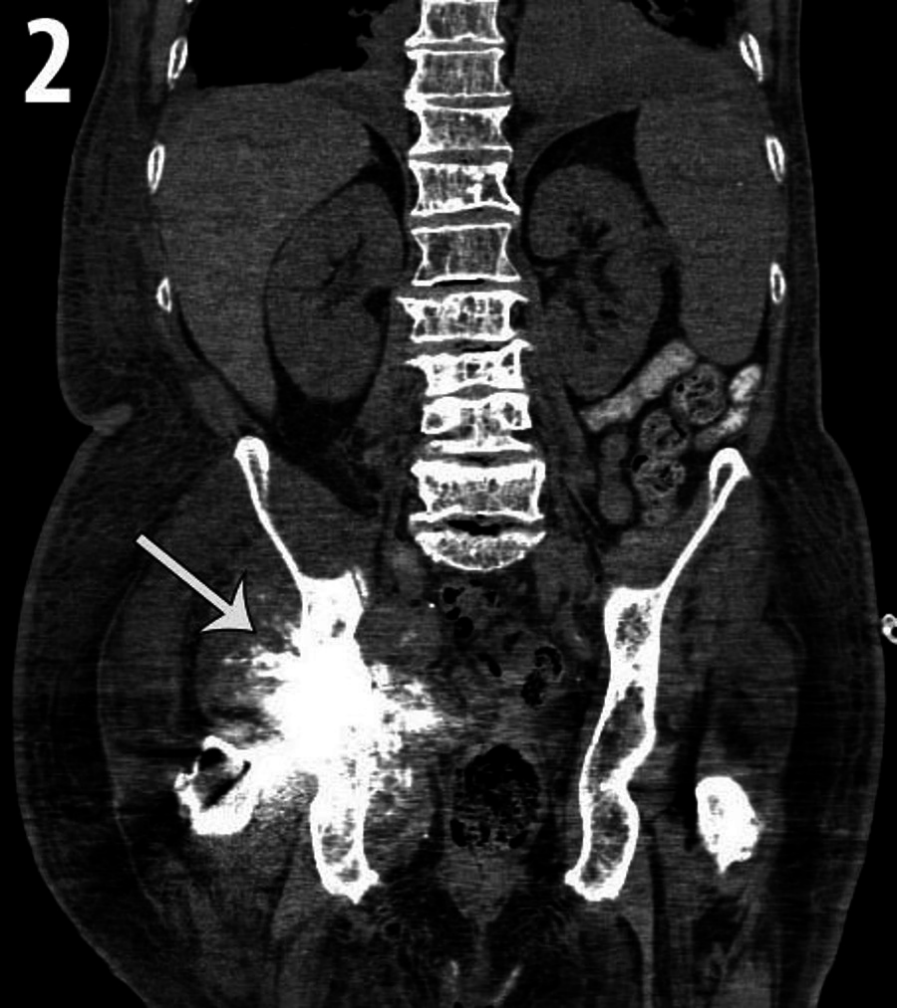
Coronal CT image of the abdomen and pelvis using soft tissue window levels. A destructive soft tissue mass is centered in the right acetabulum with a typical “sunburst” pattern of osteoid matrix (white arrow). Diffuse infiltration of the spine with myeloma is also noted. CT- Computed Tomography
